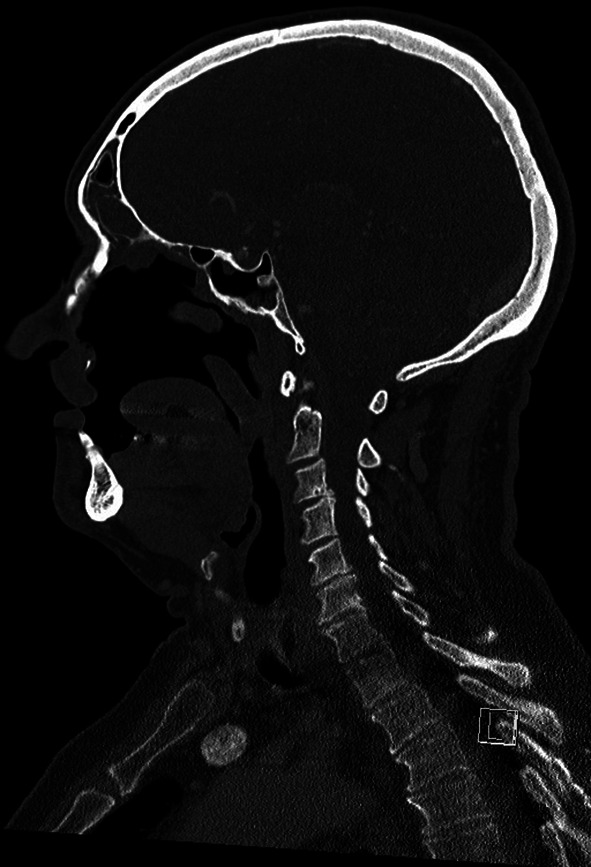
CT-scan of the head and representation of the left half of the face with missing anatomical border above the tongue (hard palate and nasal conchae) and contact with the orbit (image 11/03/2024)
